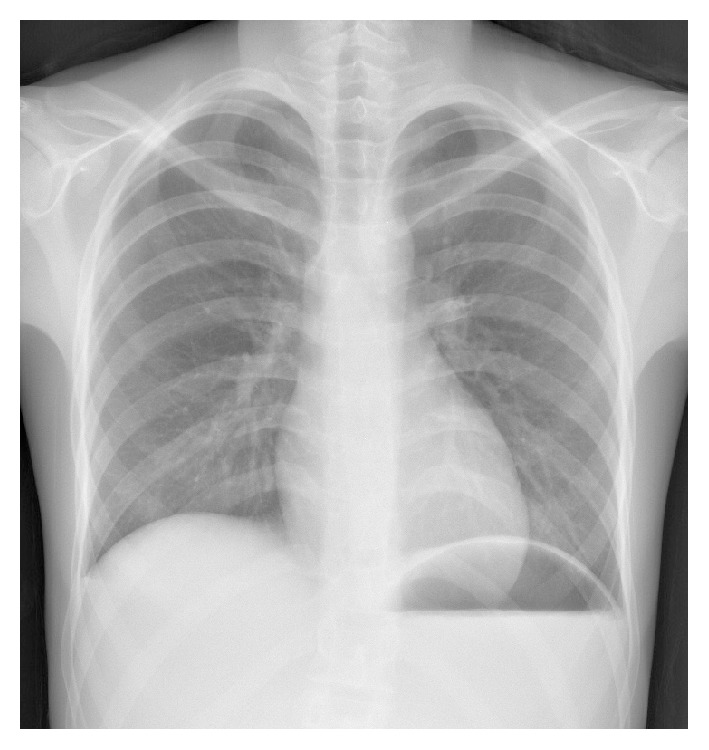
Erect chest radiograph demonstrating gastric air-fluid level.
